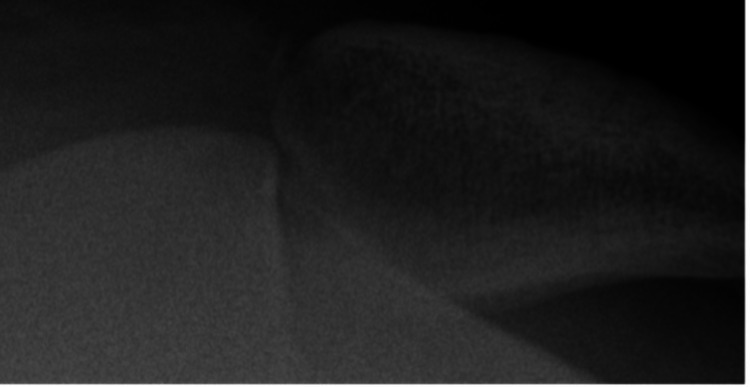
Close-up modified sunrise (patient unable to bend the knee) radiograph of the left knee showing lateral patellar dislocation with medial patellar impaction defect and avulsion fracture of the medial patella
